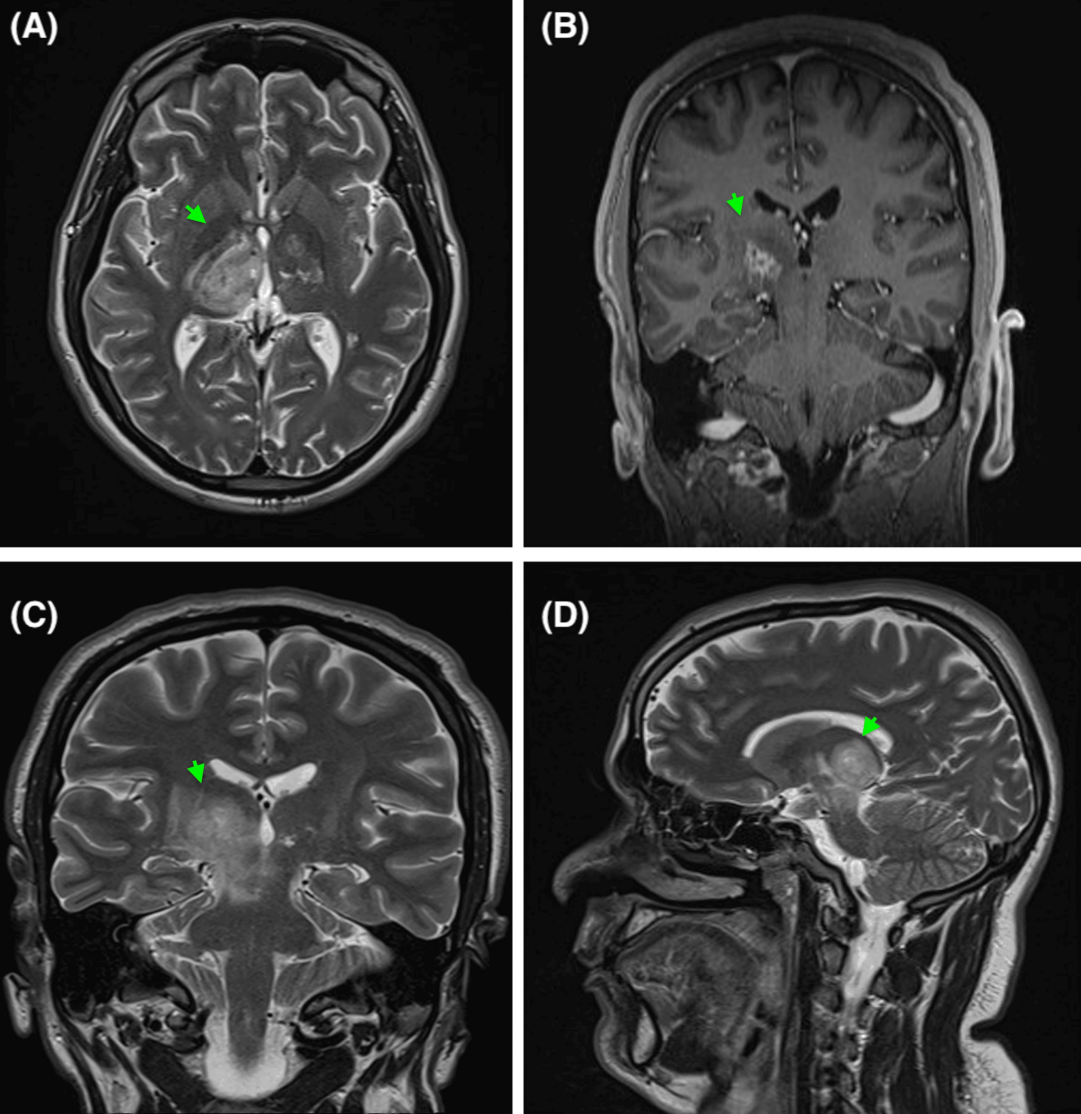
(A) Demonstrates an axial T2-weighted turbo spin echo (TSE) sequence. (B) Demonstrates a coronal T1-weighted 3D magnetization prepared rapid gradient echo (MPRAGE) post-contrast sequence. (C) Demonstrates a coronal T2-weighted TSE sequence. (D) Demonstrates a sagittal T2-weighted TSE sequence. The brain MRI demonstrates signs of significant interval regression of a previous left thalamic lesion alongside a newly developed right-sided thalamic heterogeneous enhancing lesion, and a proper aspect of the midbrain indicating disease progression.
